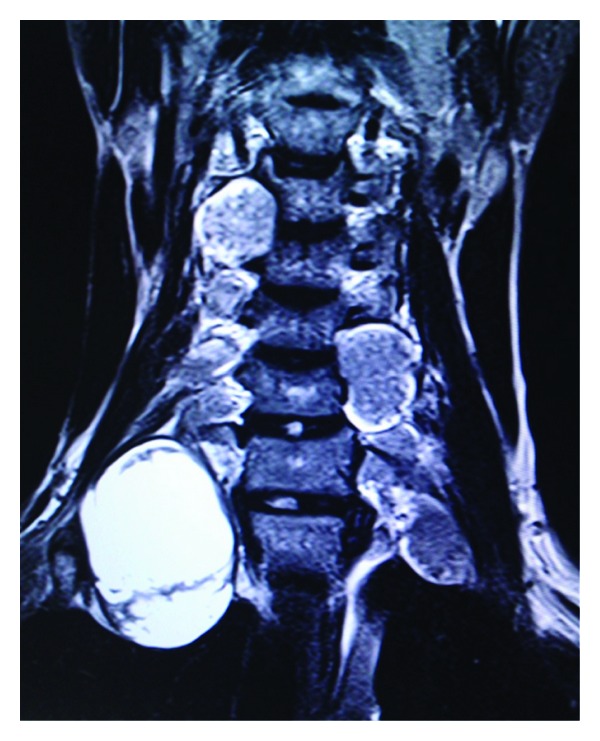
Coronal T2 weighted MR image showing multiple variable sized intra- and extradural dumbbell-shaped tumours in cervical and upper thoracic region suggestive of spinal and extraspinal neurofibromas.
